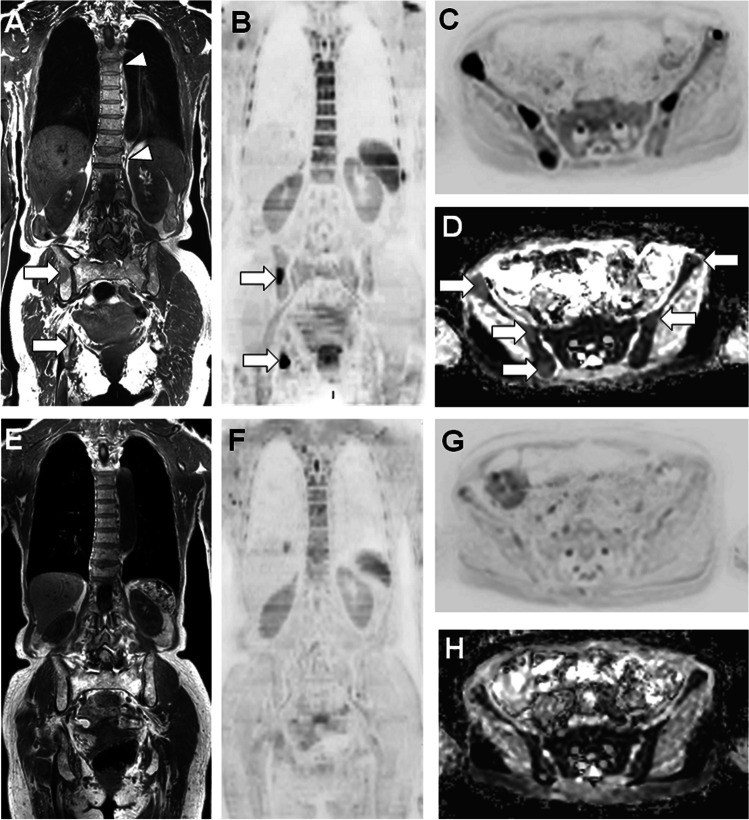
80-year-old woman with MM (IgG lambda stage IIIA, ISS 1, adverse cytogenetics (t(14;16)) – VGPR after 8 cycles of VCD): WB-MRI performed for evaluation of treatment response shows complete imaging response with no residual disease. A-B Baseline MRI findings. Coronal T1 (A) and DWI (B = 1000 s/mm^2^) (B) MR images show active bone lesions within the right pelvic region (arrows), and benign appearing vertebral fractures (arrowheads). C–D Transverse DWI (B = 1000 s/mm^2^) image (C) and ADC map (D) within the pelvis show presence of 3 lesions on the right and 2 on the left. ADC in the lesions was 750 mm^2^/s, Vs 480 mm^2^/s in the adjacent marrow. E–F Post-treatment MRI findings. Coronal T1 (E) and DWI (B = 1000 s/mm^2^) (F) MR images show disappearance of all bone lesions. G–H Transverse DWI image (G) and ADC map (H) show disappearance of pelvic lesions and return to normal of ADC values
